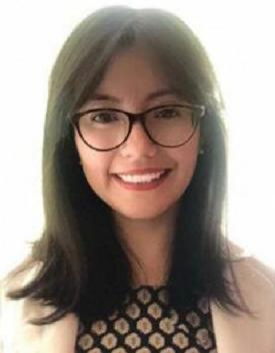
Laura Victoria Torres-Araujo is a young cardiologist working at the National Heart Institute ‘Ignacio Chávez’ in Mexico. Additionally, she serves as a CMR and CT consultant at CT Scanner Lomas Altas. Following the completion of her CMR fellowship at Royal Brompton Hospital, she is currently engaged in imaging and research endeavours within the CMR and CT field.
